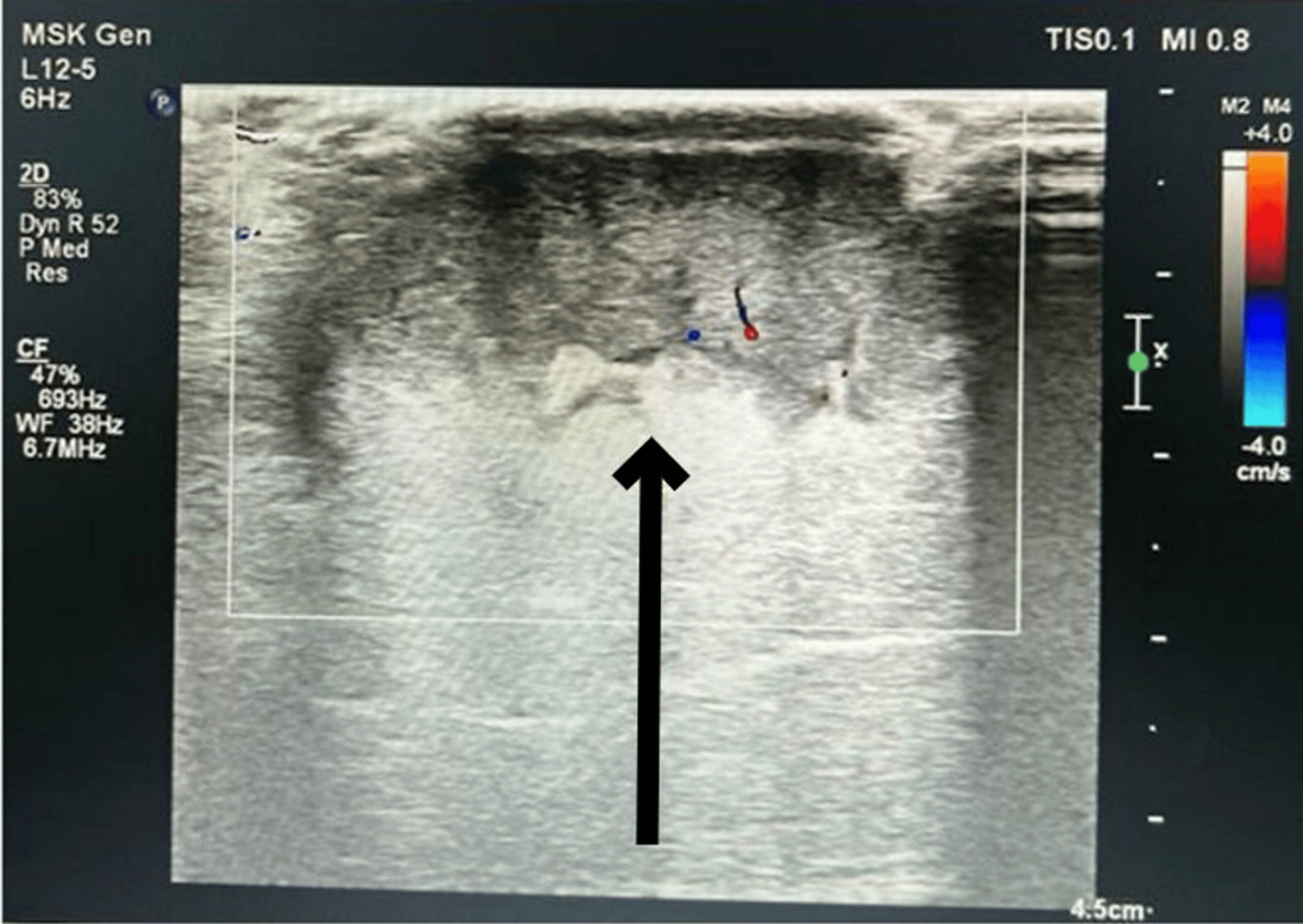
USG image: The soft-tissue swelling in the subcutaneous plane USG: ultrasonogram The black arrow shows soft-tissue swelling in the subcutaneous plane.
